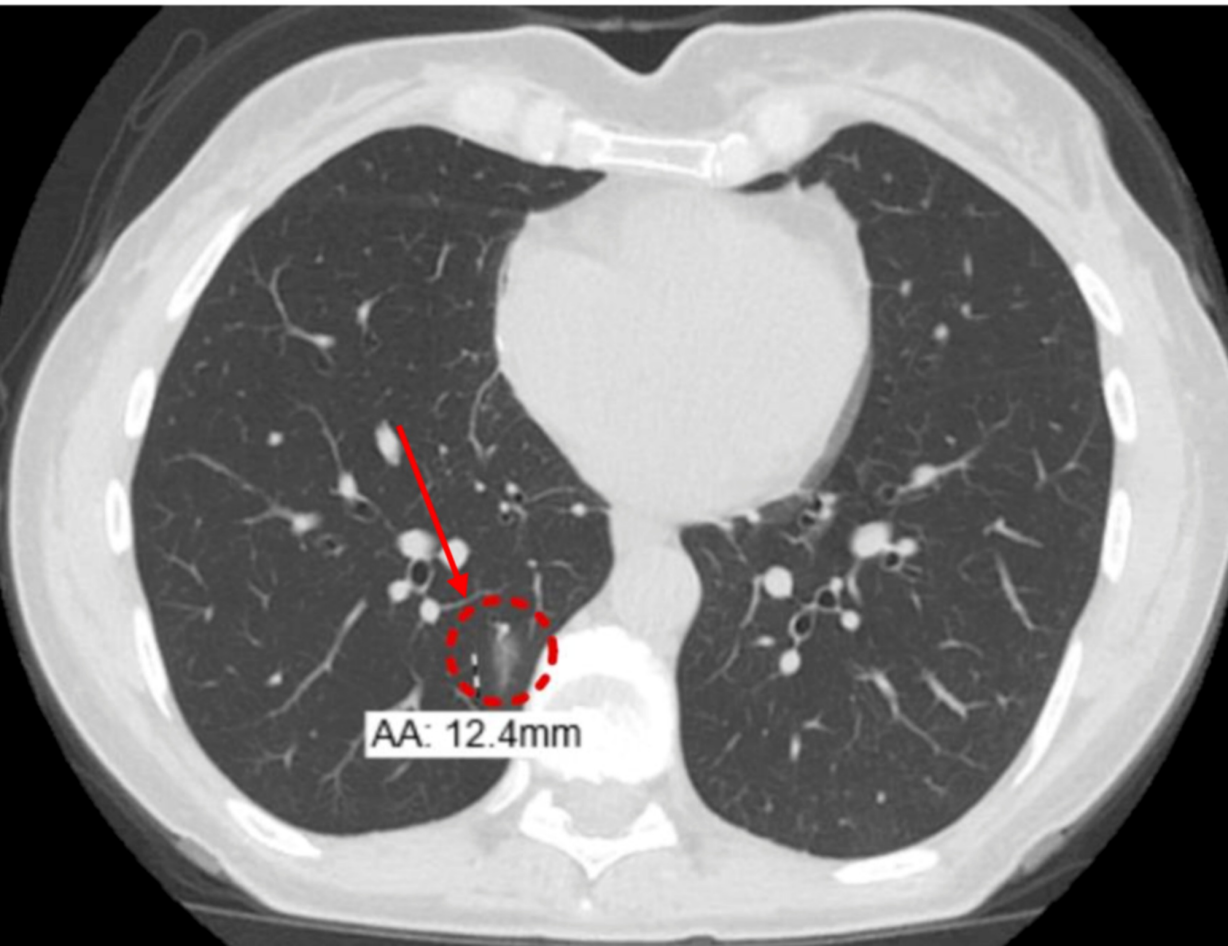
Non-contrast computerized tomography (NCCT) of the chest (eight months from previous CT) Postsurgical changes from right middle lobe resection; enlarging ill-defined 12.4 mm ground-glass opacity in the posteromedial right lower lobe
